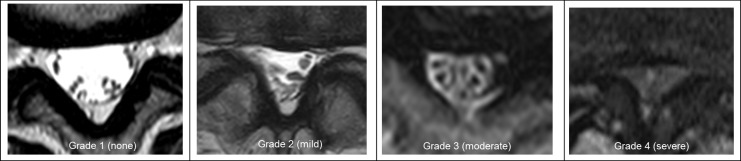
Lumbar spinal stenosis grading, based on magnetic resonance images. The general guideline classification was applied. Grade 1: mild stenosis as narrowing of the normal area by one-third or less, Grade 2: moderate stenosis as narrowing between one-third and two-thirds, Grade 3: severe stenosis as narrowing of more than two-thirds.
